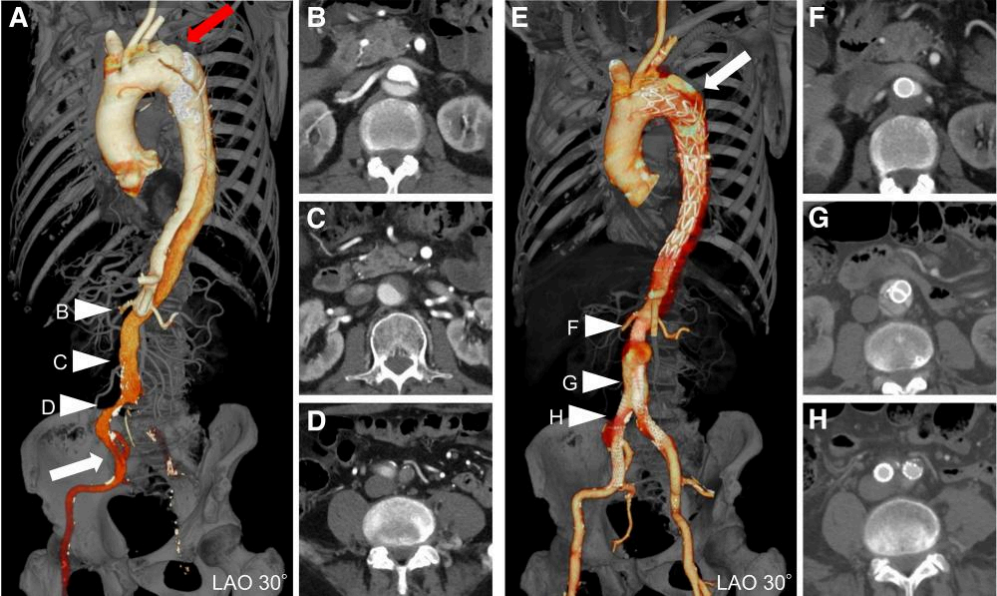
Computed tomography of pre- and post-hybrid endovascular therapy. (*A*) The large proximal entry was distal to the left subclavian artery (arrow). The true lumen of infrarenal aorta and bilateral common iliac arteries was totally collapsed by false lumen (arrowheads). The distal re-entry was distal to the right internal iliac artery (arrow). (*E*) The thoracic stent grafts were deployed (arrow) by transfemoral approach. The true lumen reconstruction by utilizing CERAB and DDMT was performed at abdominal aortic bifurcation (arrowheads).
